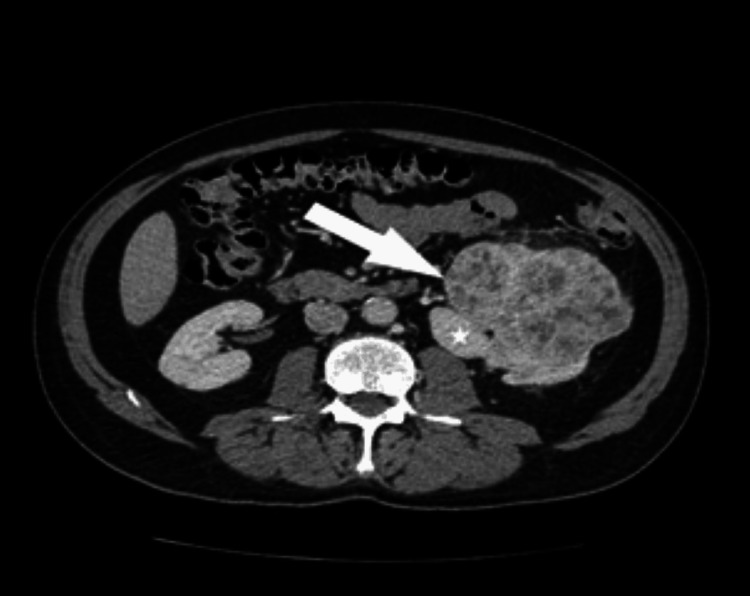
During the arterial (a) and urographic (b) phases of contrast-enhanced CT (CECT), a comprehensive evaluation unveils a considerable, expansive, and heterogenous soft-tissue lesion centrally situated within the left kidney. Notably, this lesion demonstrates invasion into the renal sinus. The heterogeneous appearance of the mass is attributable to variable enhancement patterns, with heightened enhancement evident in the periphery juxtaposed against lower enhancement centrally, which corresponds to areas of necrosis within the tumor. These characteristic features align closely with the diagnostic criteria for renal cell carcinoma (RCC). The left renal vein is marked by the asterisk at the renal sinus level. This figure is the original work of the authors. Patient consent for the use of the image was obtained, as mentioned in the patient consent form (point no. 12).
